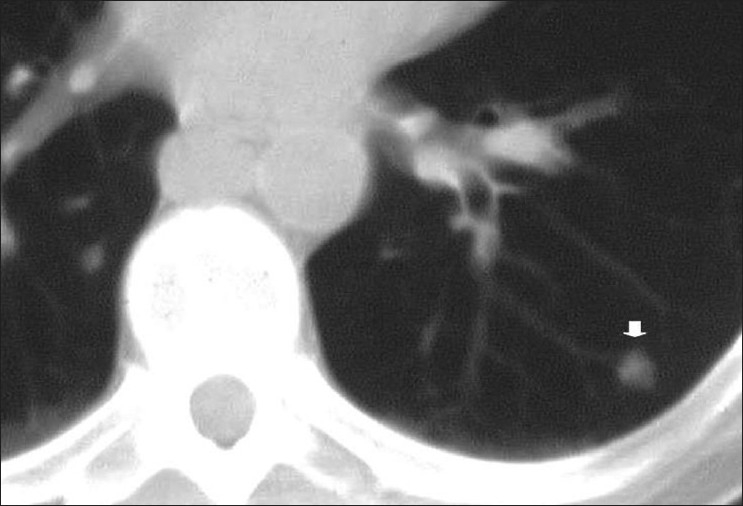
Aspergillosis. Less-common patterns include computed tomography nodules (arrow) with a peripheral halo of ground-glass attenuation (not shown)
